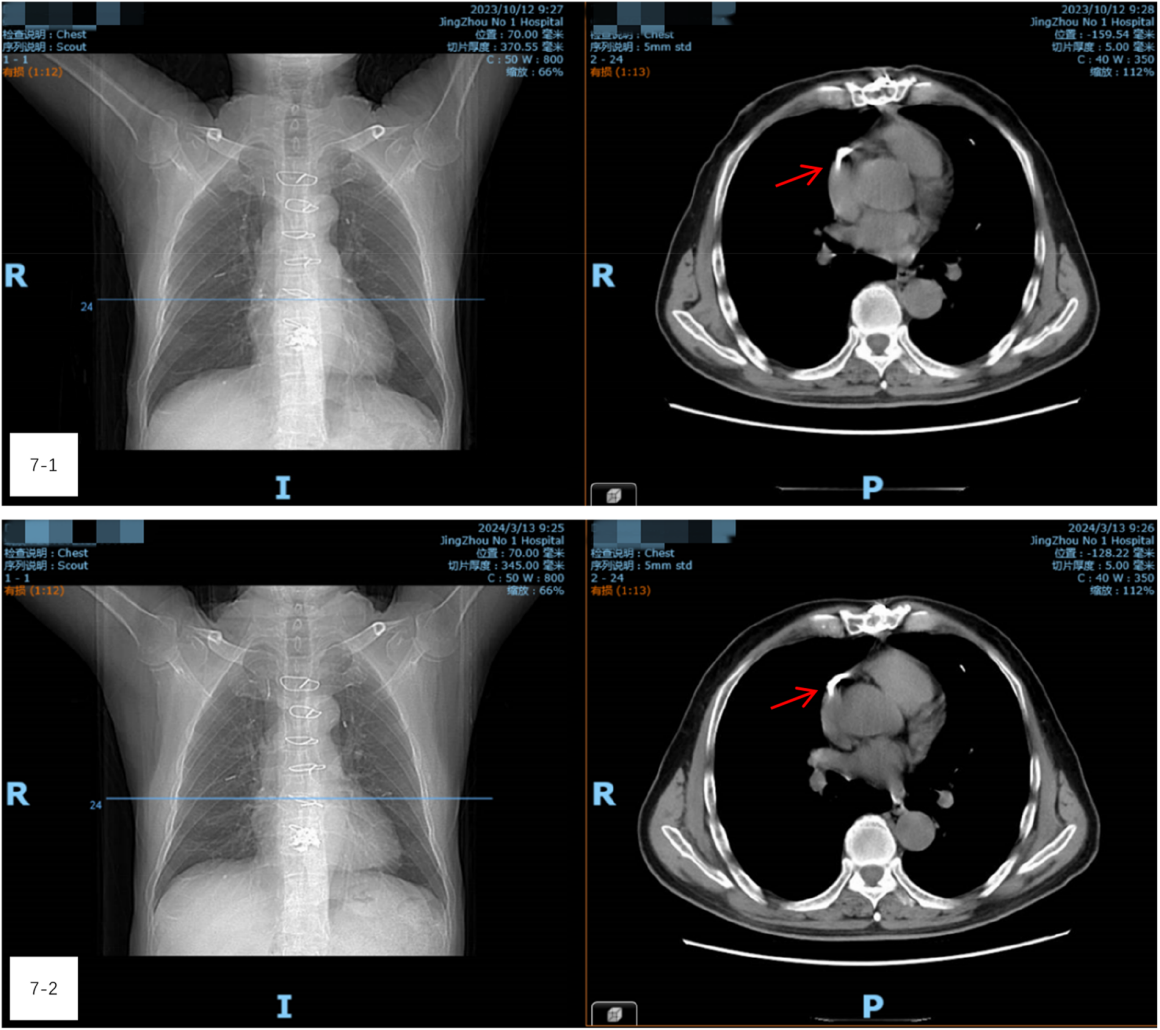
(7-1 and 7-2) Follow-up examinations one- and three-months post-surgery. CT scans showed no significant changes in the cement fragments within the heart and lungs, indicating stable conditions.
